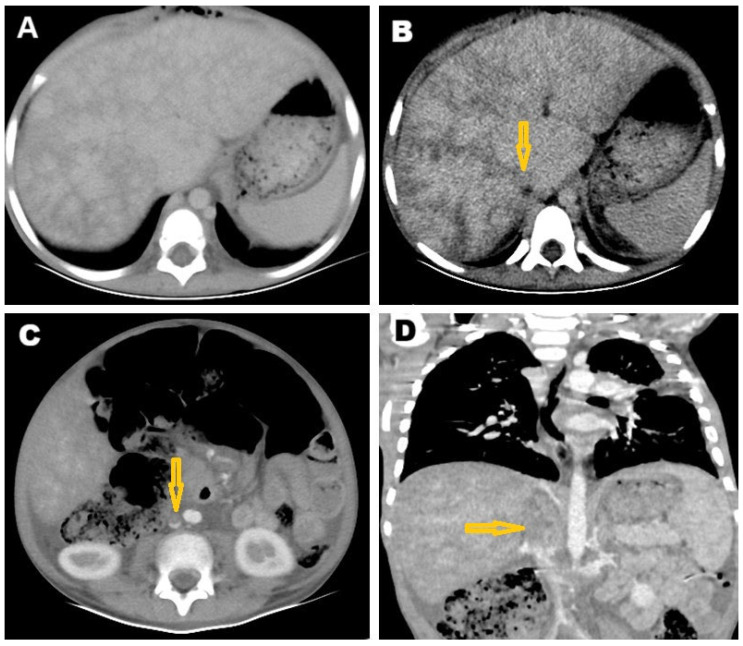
Selected images from triphasic liver computed tomography study. (A) Post-contrast venous phase axial CT of the liver showing mottled appearance of the liver due to congestion, likely Budd–Chiari syndrome; (B) post-contrast delayed-phase axial CT of the liver showing heterogeneous liver density with infiltration of the IVC (arrow); (C) post-contrast axial CT showing filling defect of the inferior vena cava (IVC) as a consequence of infiltration (arrow) down to the lower abdomen; and (D) post-contrast coronal reconstruction CT confirming IVC infiltration inside the liver (arrow).
